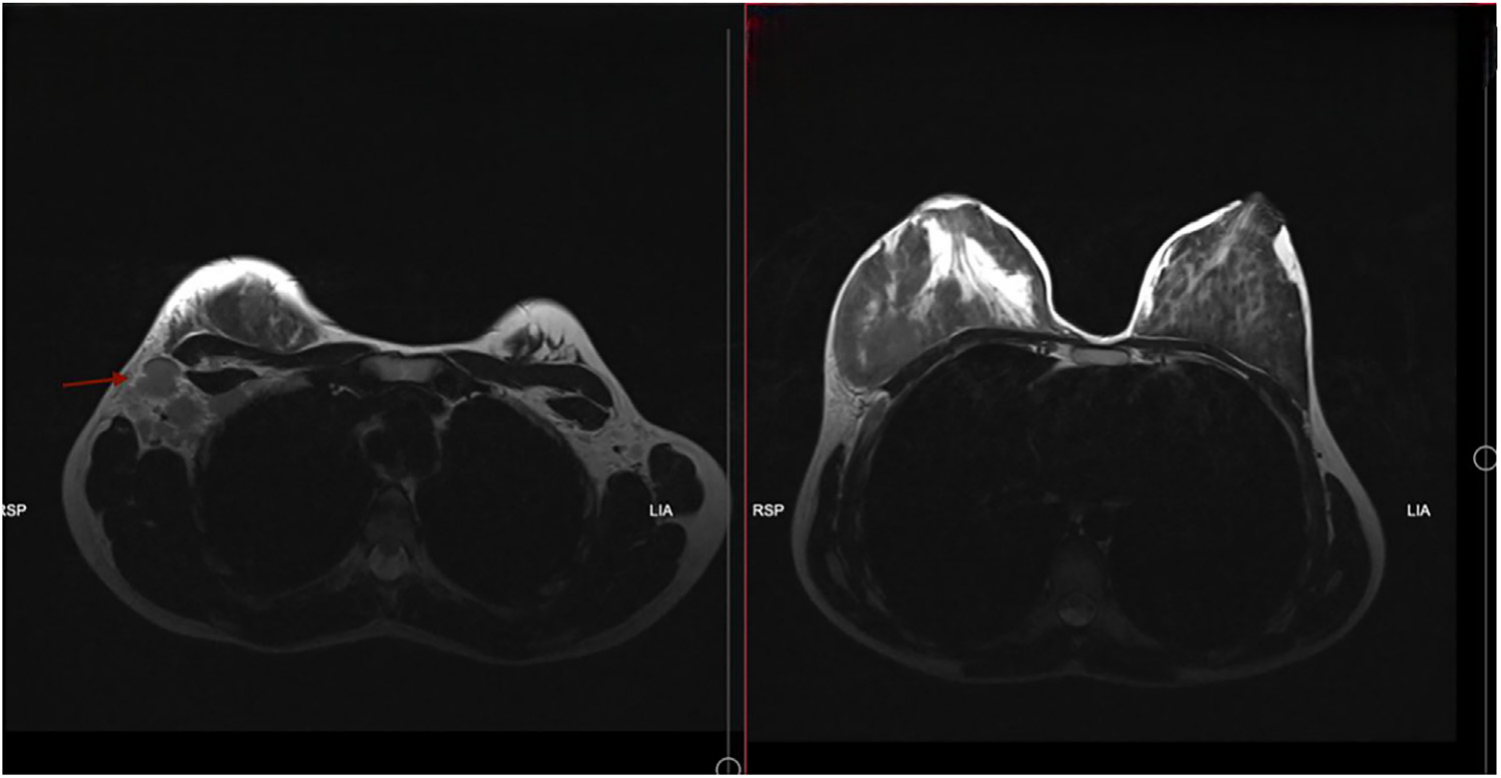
Axial view of T2 sequence of MRI of the chest.
